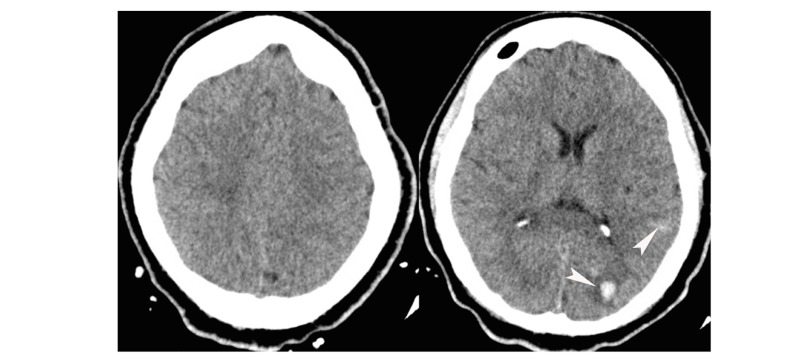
Axial non-contrast computed tomography (CT) of the head. Repeat non-contrast head CT with developing small left parieto-occipital contusions. Arrows point to developing contusions.
